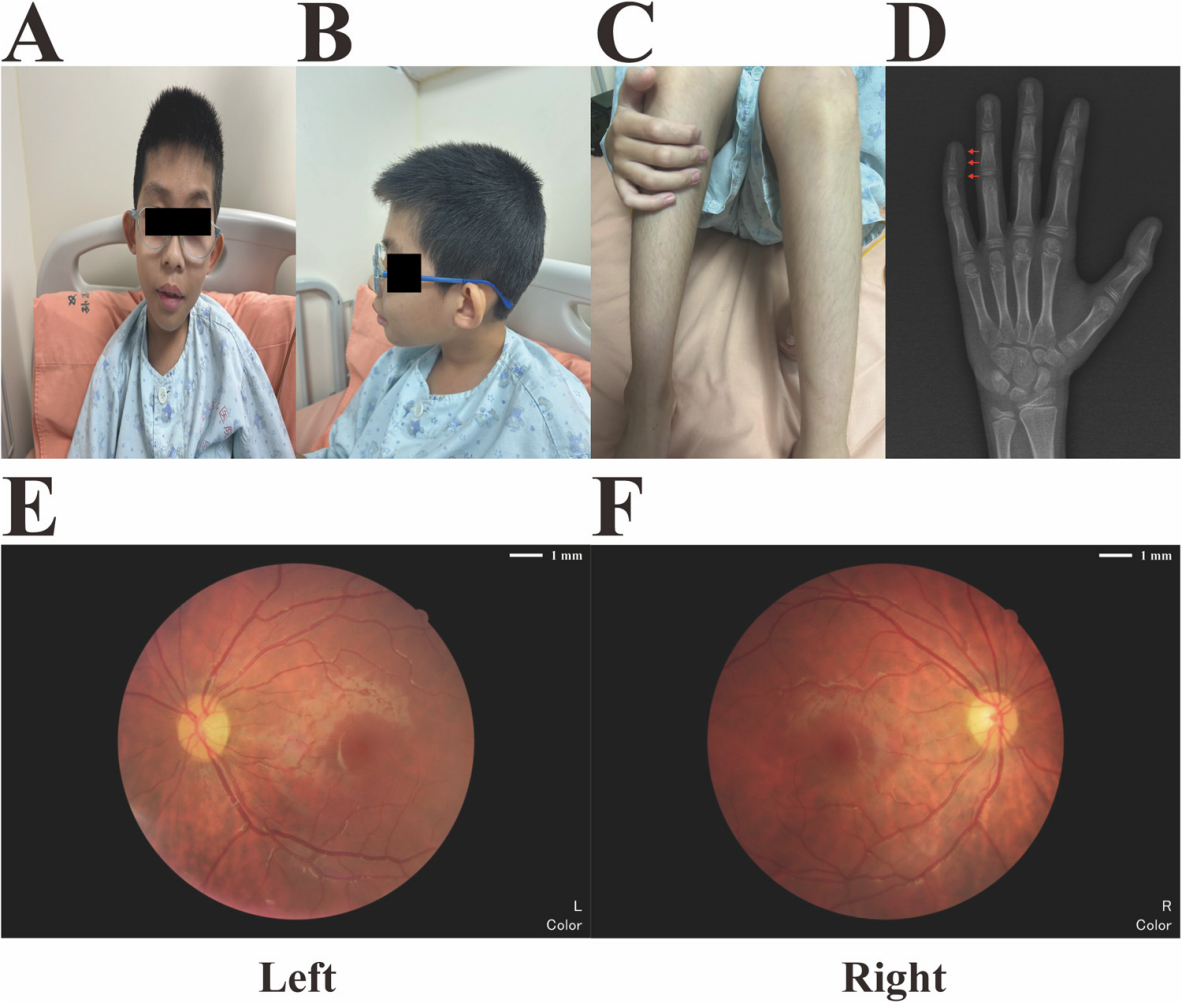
Main phenotypic characteristics of the proband. (A) Coarse facial features (thick and arched eyebrows with low-set anteriorly rotated ears) and bilateral strabismus with right eyelid ptosis. (B) Facial hirsutism and high myopia with thick-lens glasses. (C) Hypertrichosis of the proband's shins. (D) X-radiograph of the proband's left hand at the age of 10, which revealed distal phalanx hypoplasia of the fifth finger and mild clinodactyly (red arrows). (E,F) Fundus imaging revealed obvious fundus tessellation changes in both eyes of the proband.
